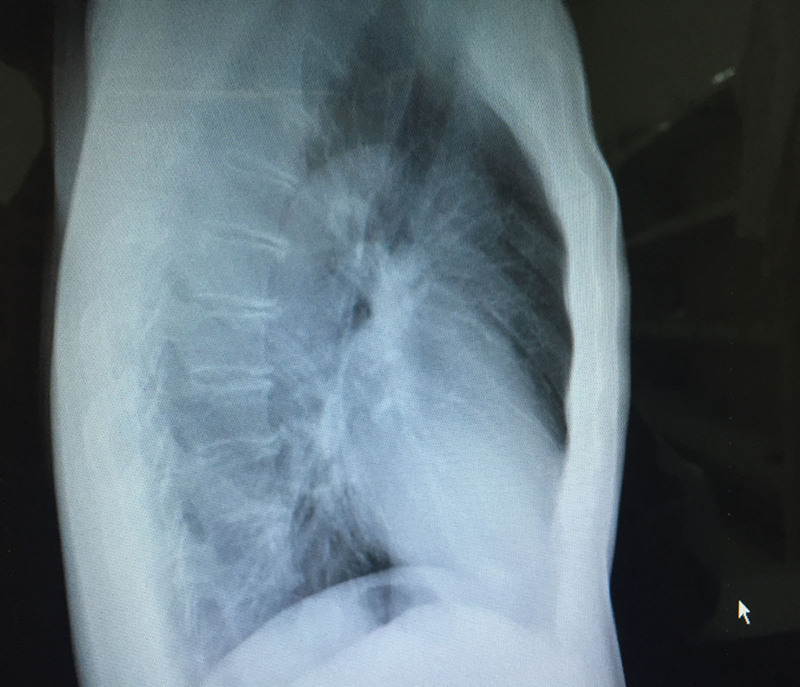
Chest X-ray displaying the high-density nodule in the medial side of the left diaphragm.
